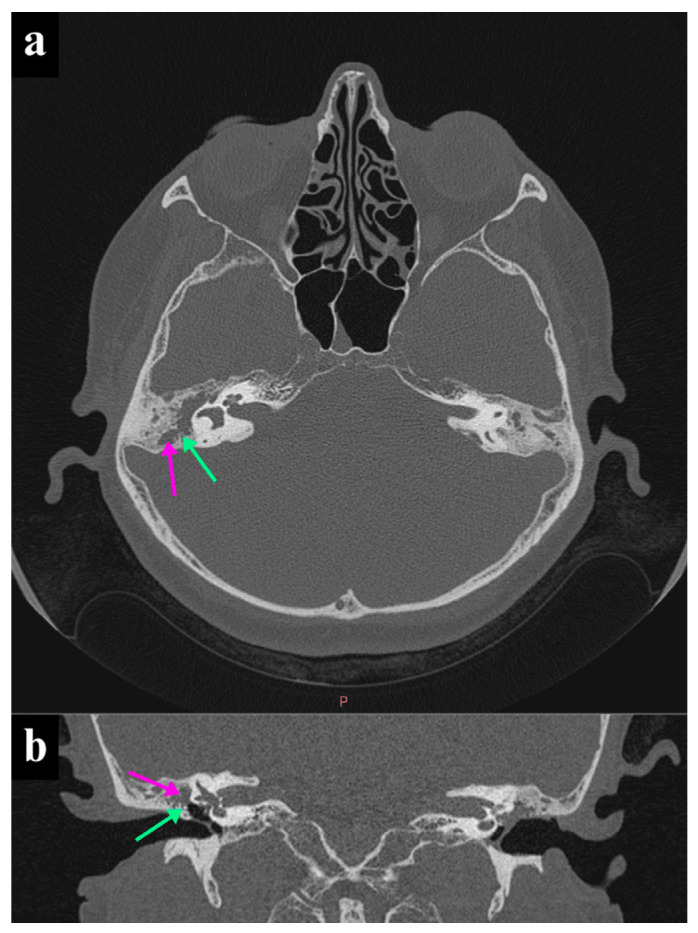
High-resolution CT of the right temporal bone showing an obstructed aditus ad antrum. (a) The axial image shows an obstructed aditus ad antrum (green arrow) and opacification of the antrum (purple arrow). (b) The coronal image again shows an obstructed aditus ad antrum (green arrow) and opacification of the antrum (purple arrow).
